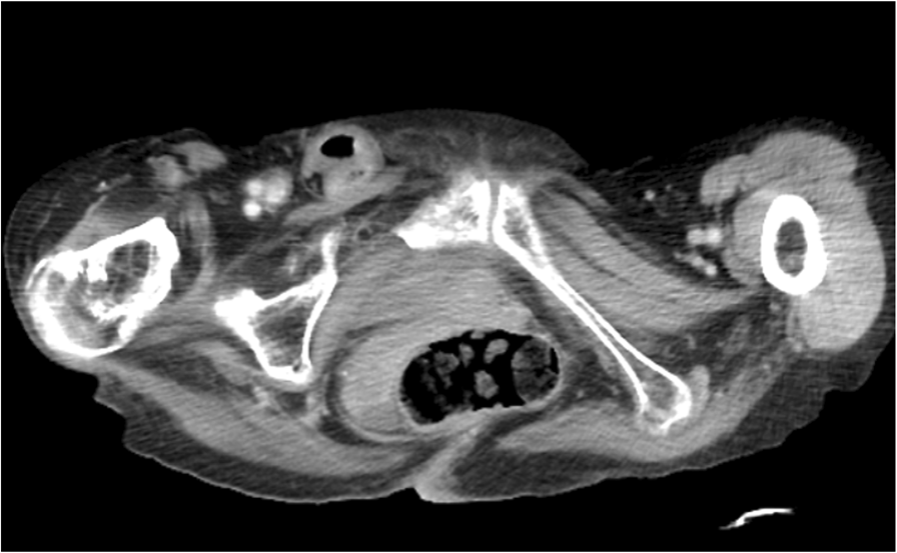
Computed tomography (CT). Enhanced CT shows a right strangulated femoral hernia. The intestinal wall shows enhancement
